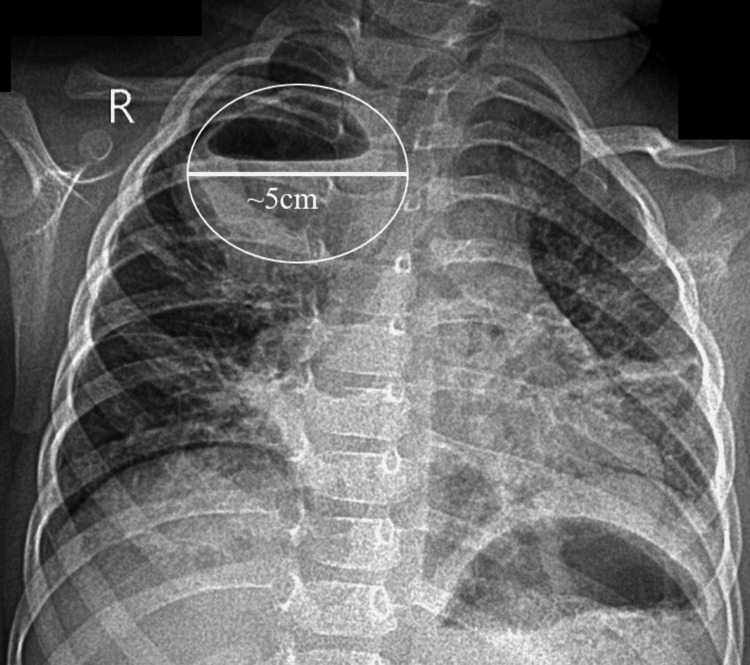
Initial Chest X-ray (CXR) Posteroanterior (PA) chest X-ray. There is a well-defined thick-walled ovoid lesion in the right upper lung (RUL) with an air-fluid level. The lesion’s max diameter is 5 cm. Also, peri-bronchial infiltrations and an atelectasis (left middle thoracic field) are present.
